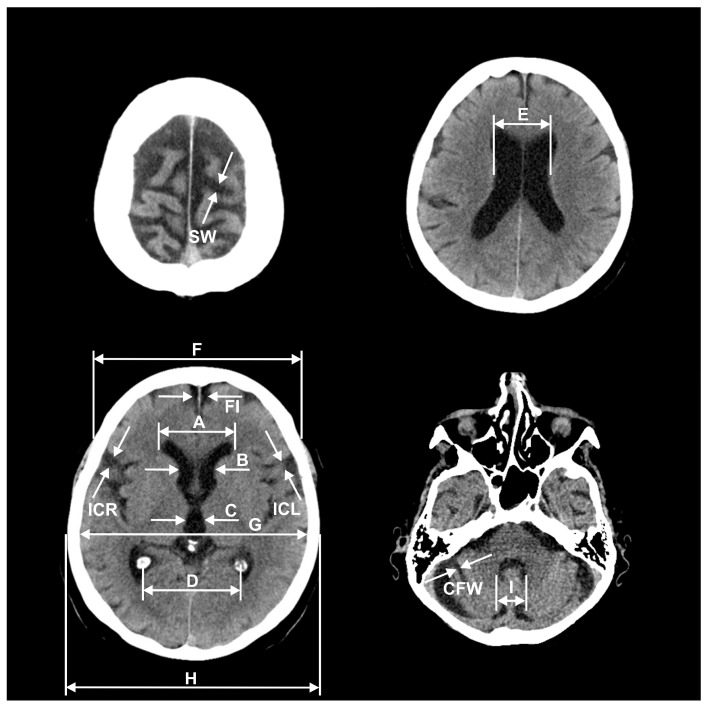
Measurements of brain atrophy parameters: A, B, C, D, E, F, G, H, I, FI, ICR, ICL, SW, CFW in CT images.
